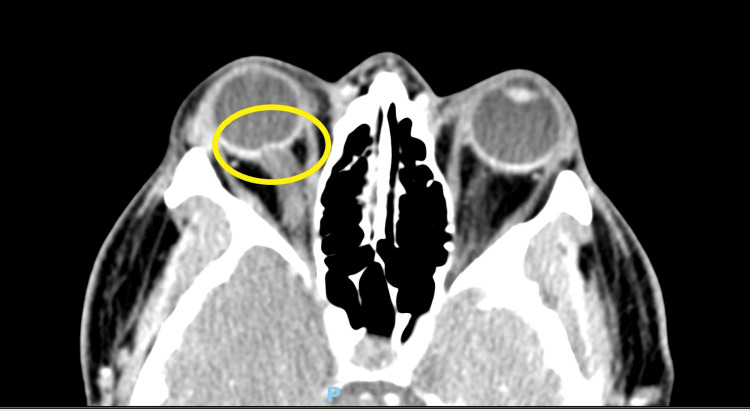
CT-head evaluating optic nerve tortuosity and posterior optic globe Yellow circle: CT-head demonstrating tortuosity of the optic nerves and flattening of the right posterior globe with probably invagination of the optic nerves through the optic cup
